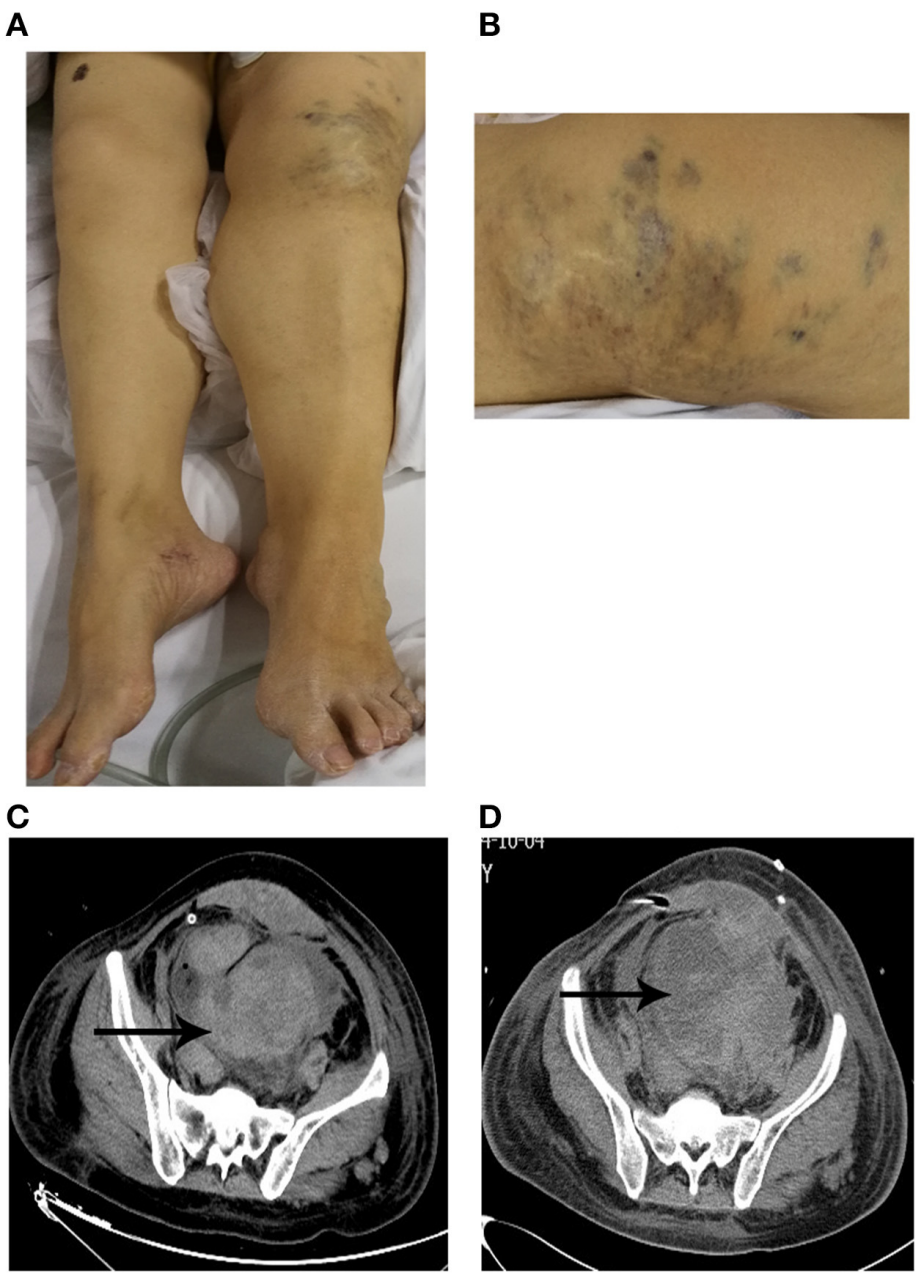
(A,B) Patient with Klippel–Trenaunay syndrome. (A) The left leg was larger in circumference and longer in length than the right leg. (B) Capillary malformation in the left leg. (C,D) Unenhanced computer tomography (CT) showed peritoneal diffuse effusion in pelvis with different mixed attenuation values at the admission to our department (C) and post-operative period (D).
